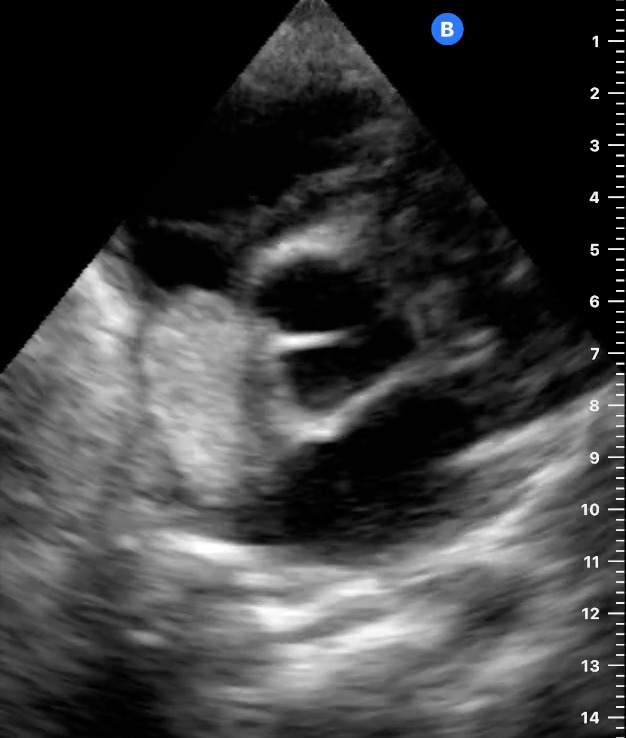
Parasternal short-axis view at the level of the cardiac base showing the right atrial mass (arrow).
